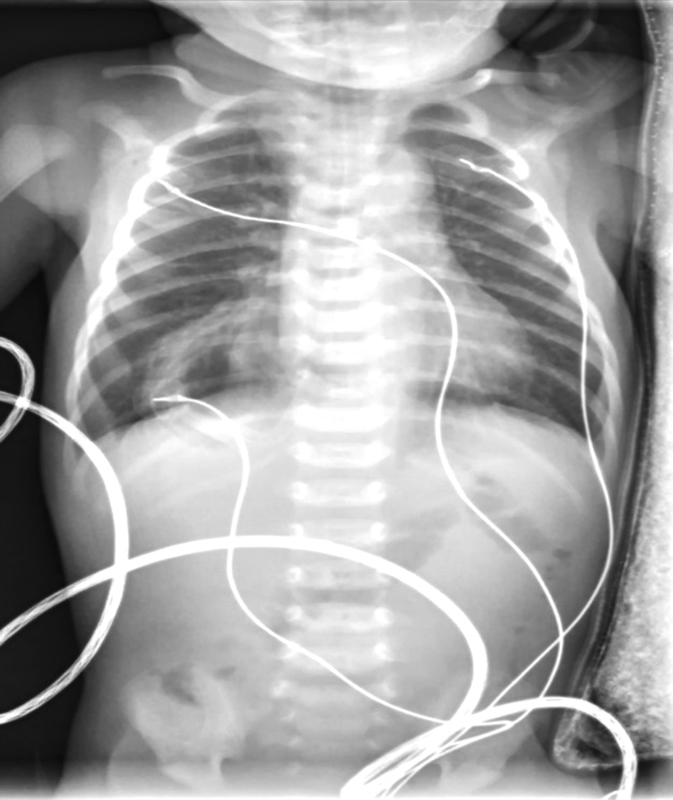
Chest X-ray showing an intrathoracic gas bubble compatible with stomach.
